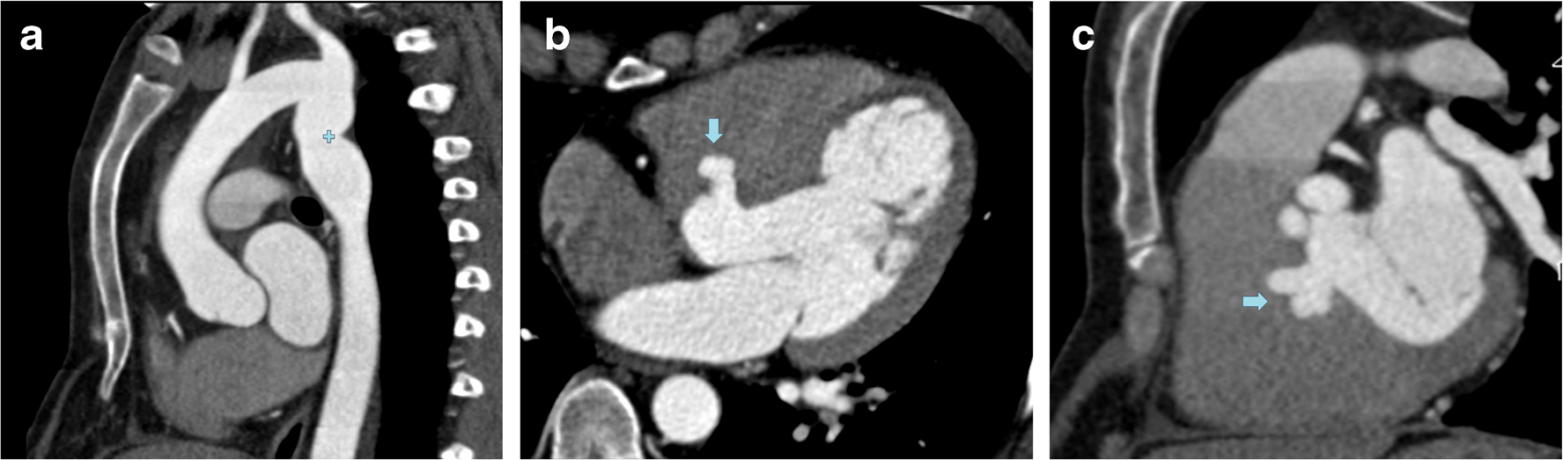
33-year-old man with pectus excavatum deformity and aortic pseudocoarctation. MPR of the aortic arch (a) shows an aortic indentation (+) at the level of the proximal descending aorta. Axial (b) and coronal oblique MPR (c) CT images show a lobulated IVMS aneurysm (*arrows)* projecting into the base of the right ventricle
